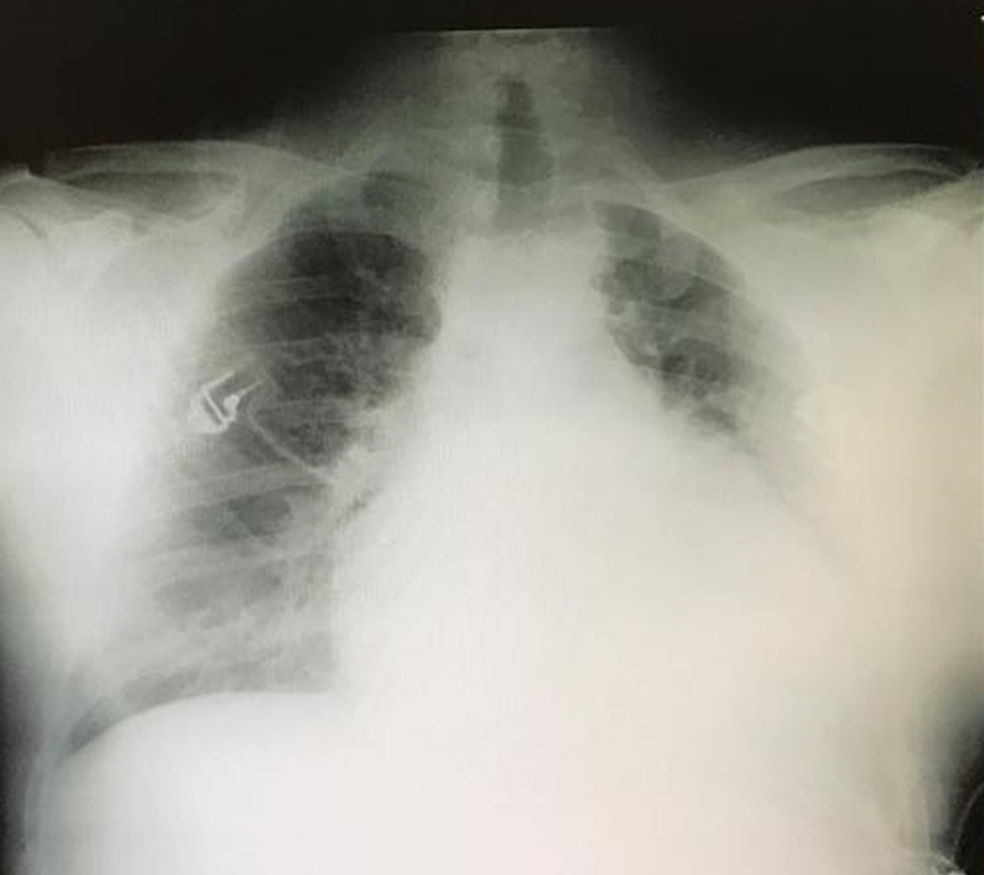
Plain radiography. Globular enlargement of the cardiac shadow giving a water bottle configuration.
